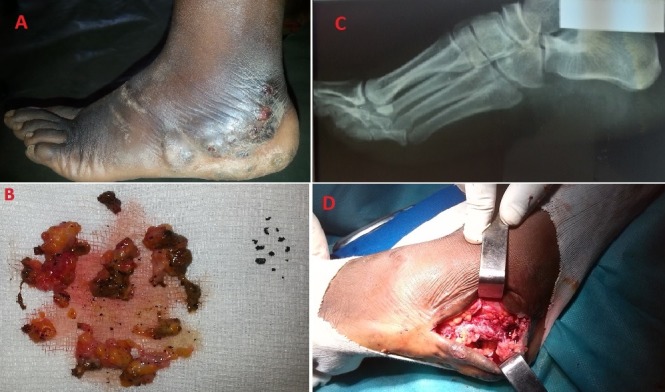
(A) tumefaction of the left foot discharging black grains; (B) black eumycetoma grains; (C) standard X-rays showed no bone lesion; (D) surgical debridement
